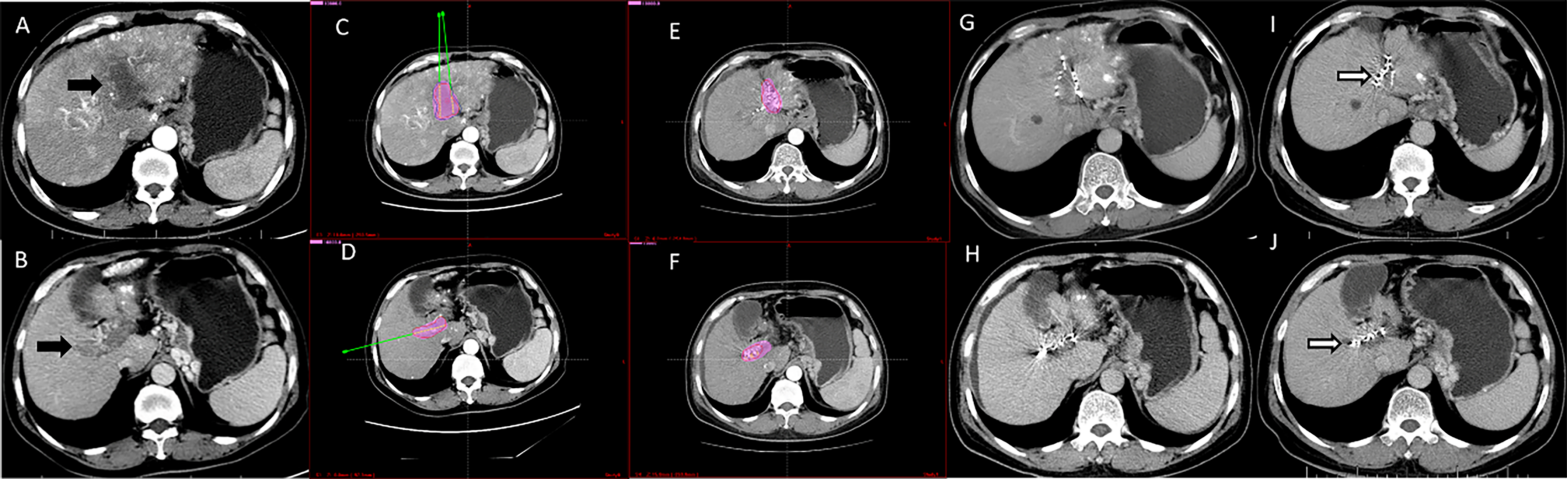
Computed tomography imaging of a 56-year-old man with hepatocellular carcinoma complicated by PVTT (Type IIb). (A, B) Enhanced computed tomography imaging showed that the thrombus had originated from the left and right branches of the portal vein. (C, D) The image prior to iodine seed implantation showed the dosage distribution of seeds in the left and right branches according to the TPS. (E, F) The iodine seed implantation was performed in left and right branches during one procedure, with the dosage verification image showing iodine seed implantation according to the treatment planning system. (G, H) Computed tomography imaging demonstrated that the PVTT had decreased in size, but the portal vein still showed obstruction at 3 months after seed implantation. (I, J) Twelve months after the procedure, the PVTT had shrunk more; however, there were no signs of recanalization. This patient died due to liver failure at 16 months after the procedure.
